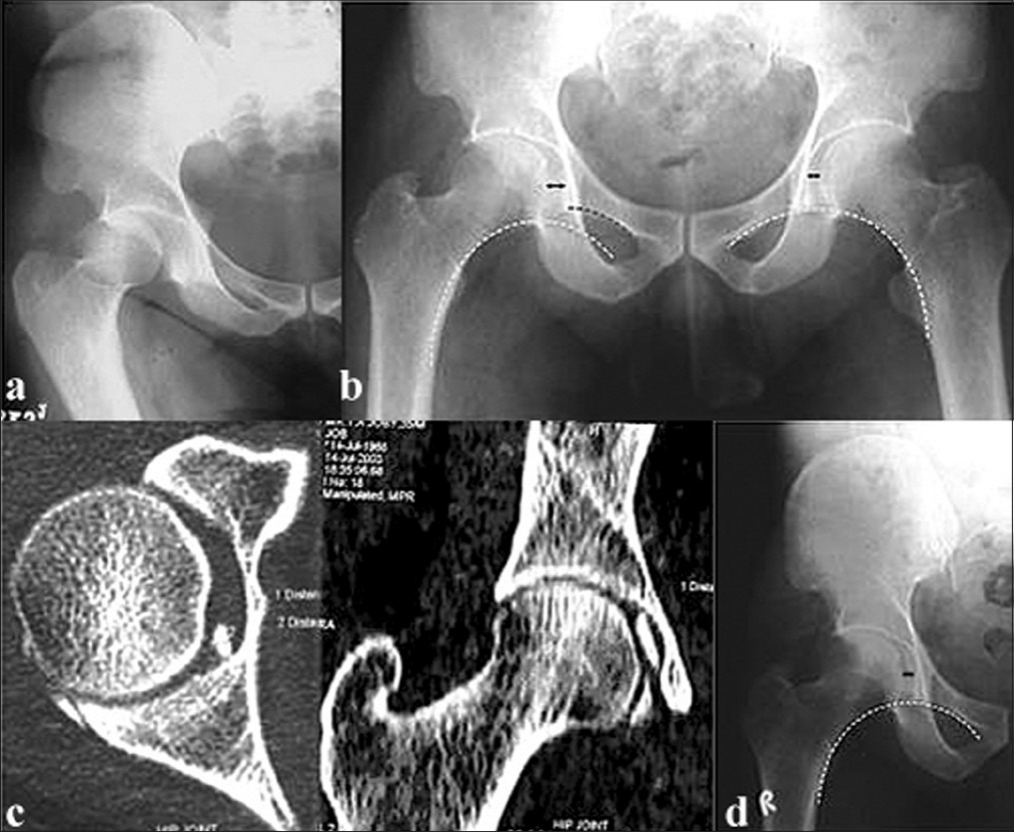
(a) X-ray of right hip joint (an anteroposterior view) showing posterior dislocation of hip in a 44-year-old male. (b) Postreduction X-ray (an anteroposterior view) shows noncongruent reduction: there is a broken Shenton’s line and increase in medial joint space. (c) CT scan shows the presence of an osteochondral fragment on the medial side of the joint. This was removed by open reduction. (d) A postoperative X-ray shows congruent reduction. The origin of the loose body was from the posterior lip of the acetabulum
